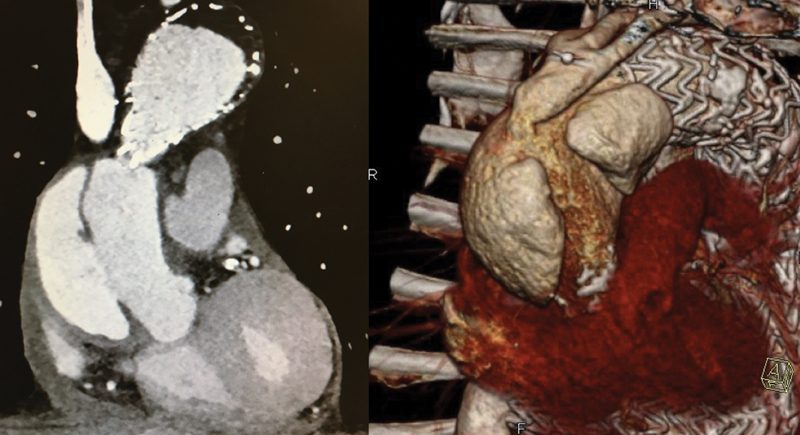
Computed tomographic angiogram showing the presence of two lumens in the ascending aorta with the three-dimensional (3D) reconstruction.
